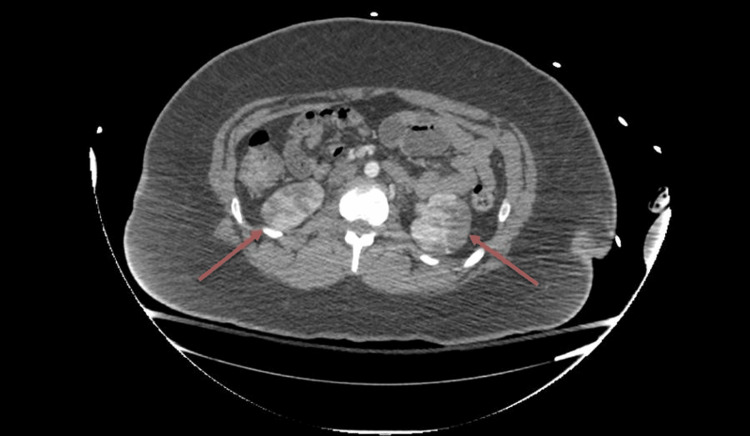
Contrast-enhanced axial-view CT scan also demonstrating bilateral renal infarcts The red arrows point to the right and left infarcted kidneys on the axial-view CT scan
